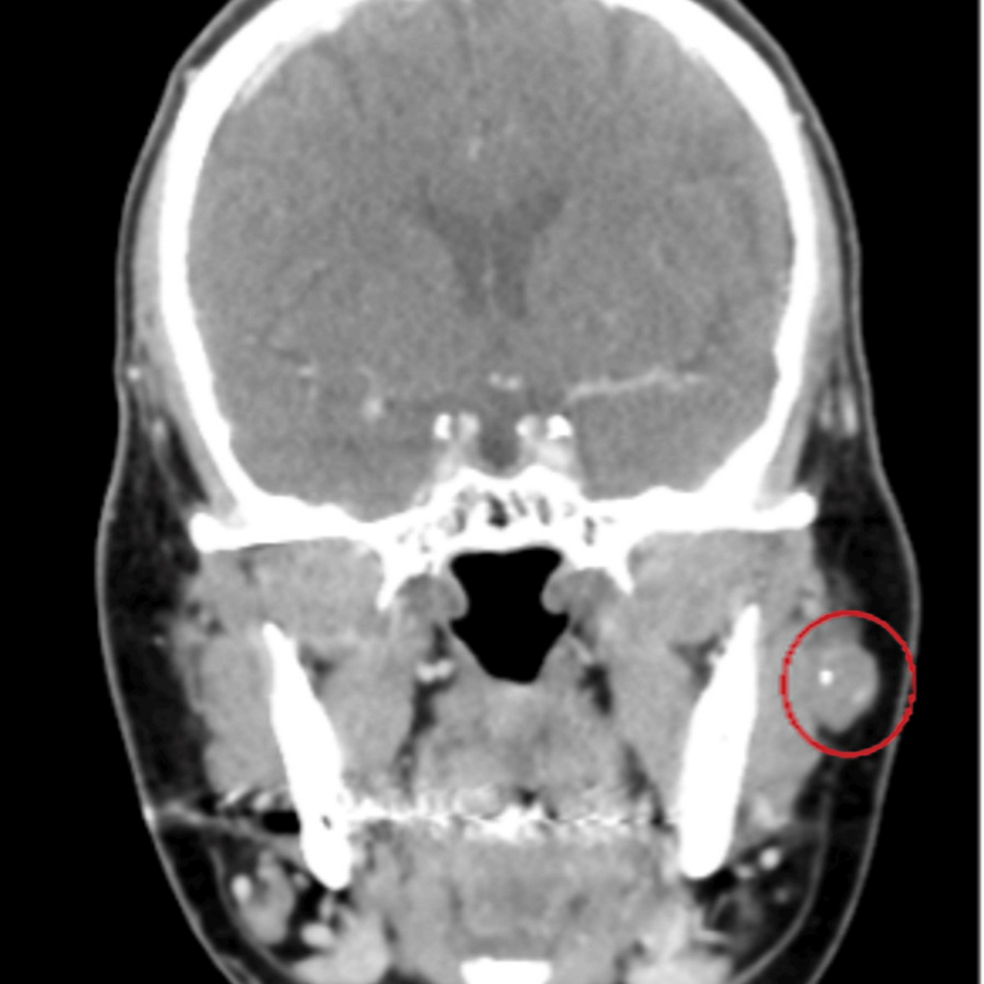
CT head and neck (coronal section) An enlarged, non-enhanced oval shaped left mandibular mass located superficial to and lying on the left masseter muscle measuring 1.2 cm with foci of calcification; minimal peripheral enhancing components.
